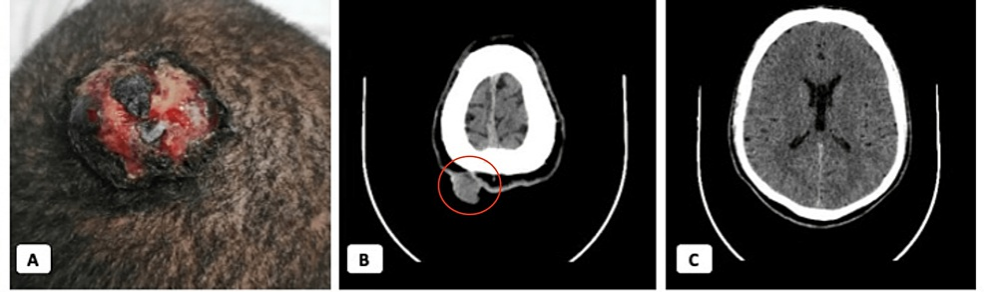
Clinical appearance of the lesion and imaging. (A) An exophytic mass located in the coronal region of the scalp, with bleeding stigmata. (B) Contrast tomography (CT) of the skull showing an exophytic mass on the scalp of the right parietal region with deep subgaleal involvement. The involved area is marked with a red circle. (C) No periosteal infiltration or intracranial involvement was observed.
